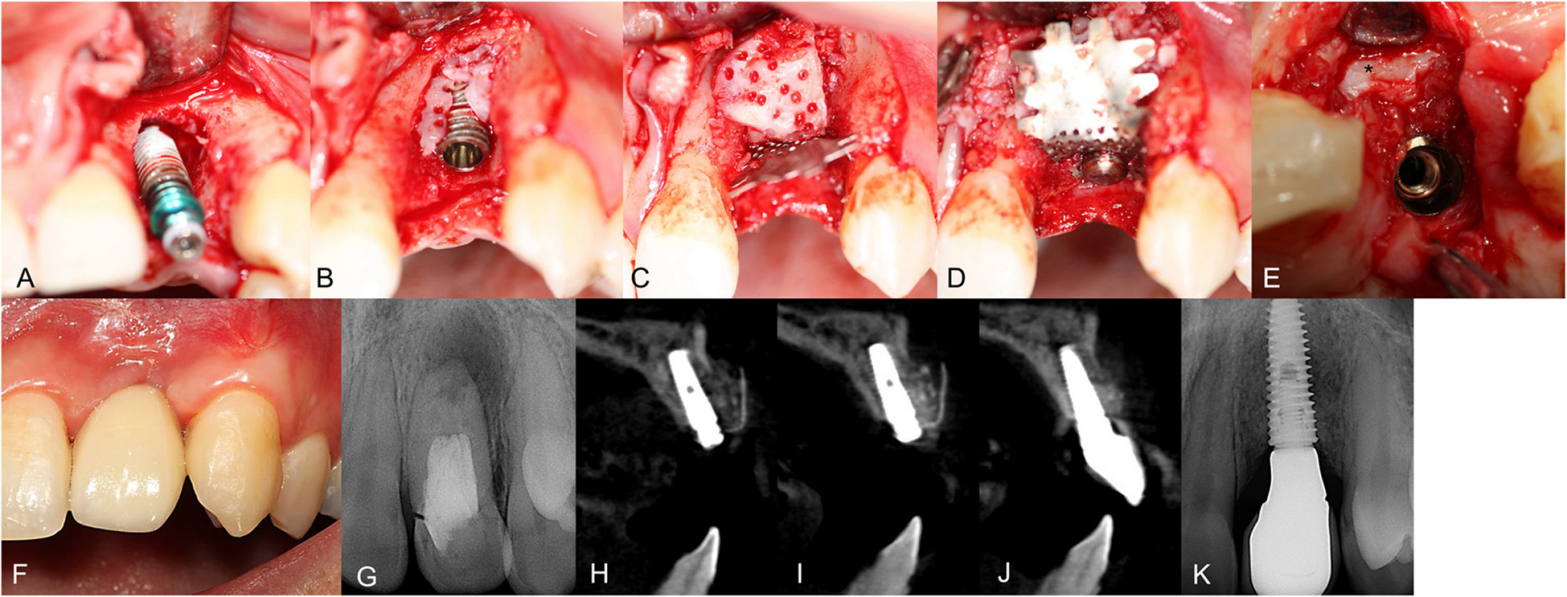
Clinical case of autogenous fresh demineralized tooth (auto-FDT) graft to support dental implant placement in a 23-year-old male. A. A large labial dehiscence remains after fixture placement in the socket of the maxillary left lateral incisor. B. The processed tooth chips are grafted for intimate contact with the fixture. C. The entire defect is covered with the block. D. A thin titanium sheet (CTi-mem™, Neobiotech, Seoul, Korea) is used to hold the graft. E. An uncovering procedure is performed 5 months after grafting. The tooth block is observed tightly fixed to the host bone. F. Five months after grafting, the final prosthesis is placed. G. A periapical radiograph shows the periodontal and periapical lesions around the lateral incisor. H–J: Series of cone beam computed tomography images. Immediately after grafting (H). After 5 months, an increase in radiopacity and decrease in volume of the graft indicate bone remodeling (I). Twelve months after final prosthesis placement, the regenerated bone provides good maintenance and support for the implant (J). Intraoral radiography obtained 12 months after final prosthesis placement. K. Intraoral radiography obtained 12 months after final prosthesis placement.
